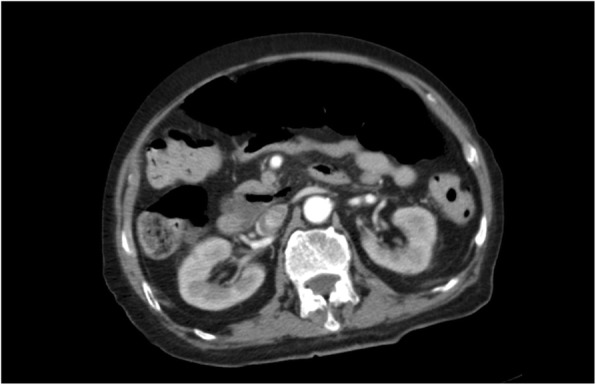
Abdominal CT scan showed colonic dilatation with no sign of thromboses and mechanical obstruction
